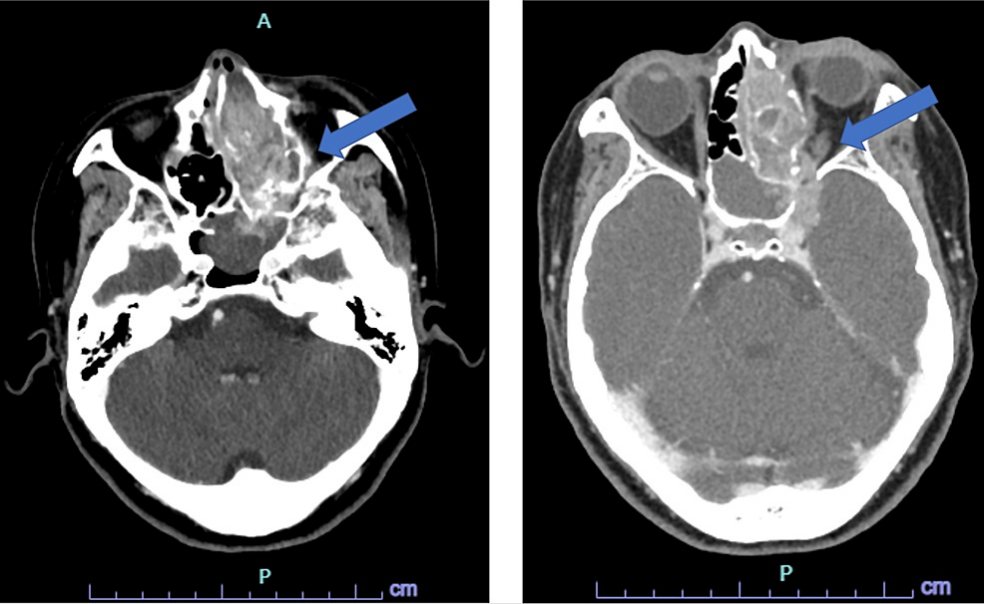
Contrast-enhanced CT imaging depicting opacification of left paranasal sinuses extending into cranium (arrow) with severe osseous erosion.
